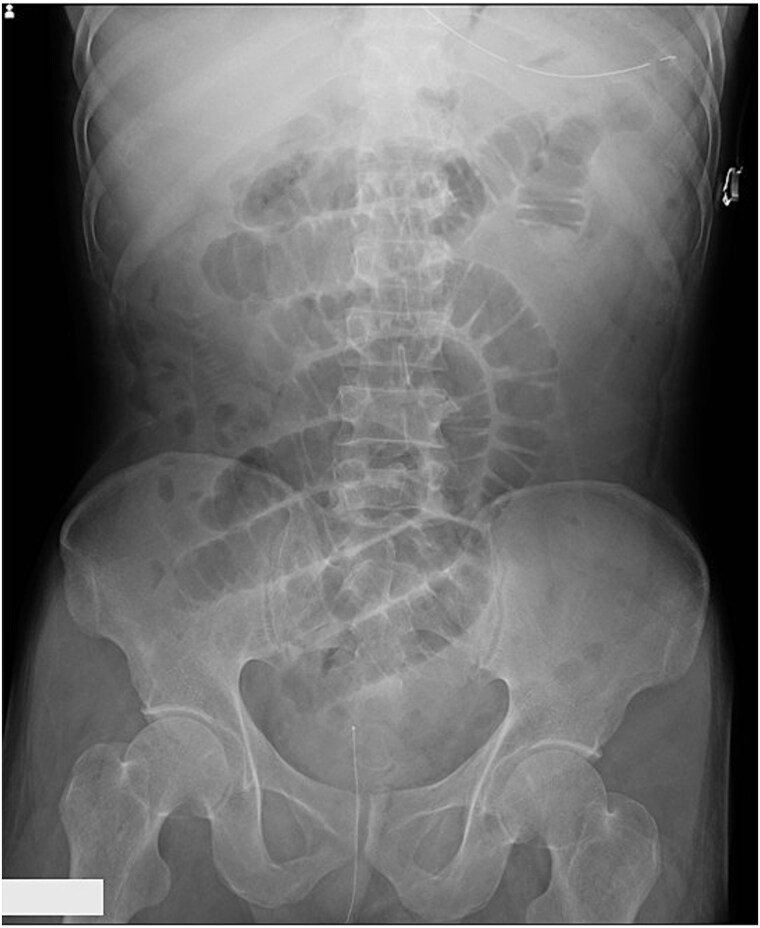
Supine abdominal X-ray showing dilated small bowel loops.
